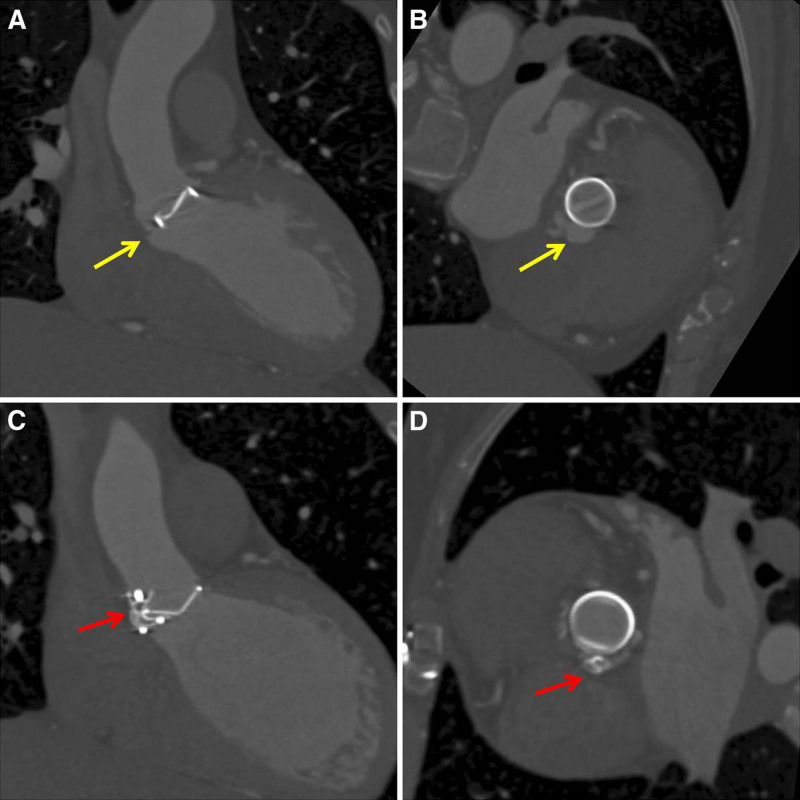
Computed tomography angiography before the procedure and during the follow-up period. (A) The aortic PVL before the procedure, sagittal view. (B) The aortic PVL before the procedure, axial view. (C) The aortic PVL was closed using the occlude, sagittal view. (D) The aortic PVL was closed with the occlude, axial view (the *yellow arrow* points to the PVL. The *red arrow* indicates the occluder). PVL = percutaneous paravalvular leak.
